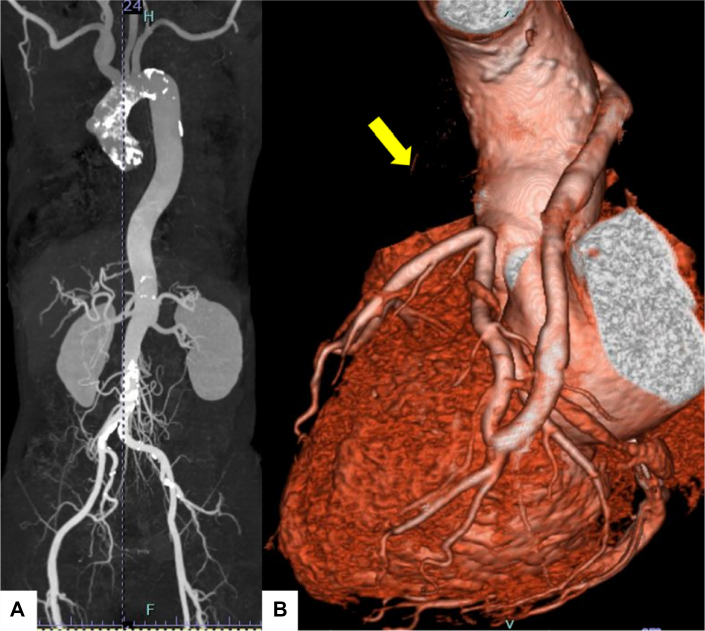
Imaging of Patient 2 (A) Computed tomography revealed circumferential aortic calcification extending from the aortic root to the descending and abdominal aorta, as well as 50% stenosis of the left common carotid artery. (B) Coronary computed tomography angiography revealed patency of the saphenous vein graph (SVG) in the obtuse marginal branch, whereas the SVG in the left anterior descending artery was occluded (yellow arrow).
